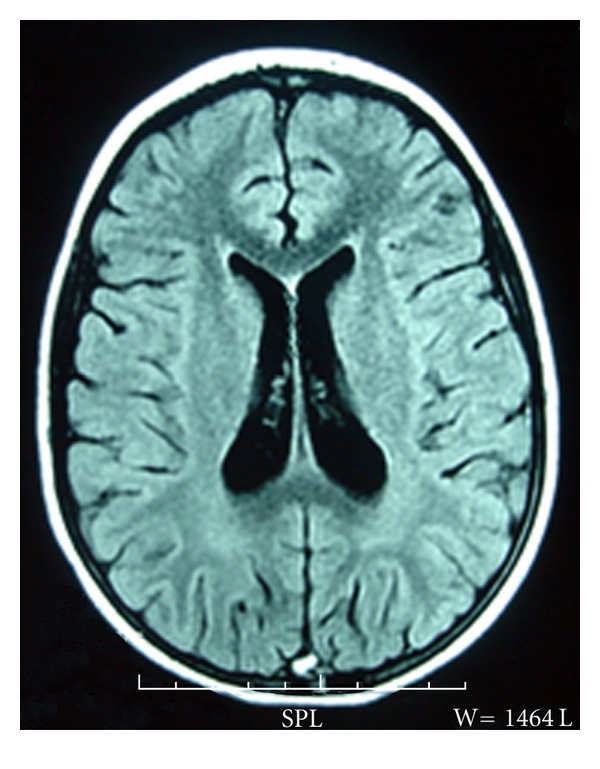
MRI of the brain (FLAIR) showed a high signal in the posterior part of the sagittal sinus.
